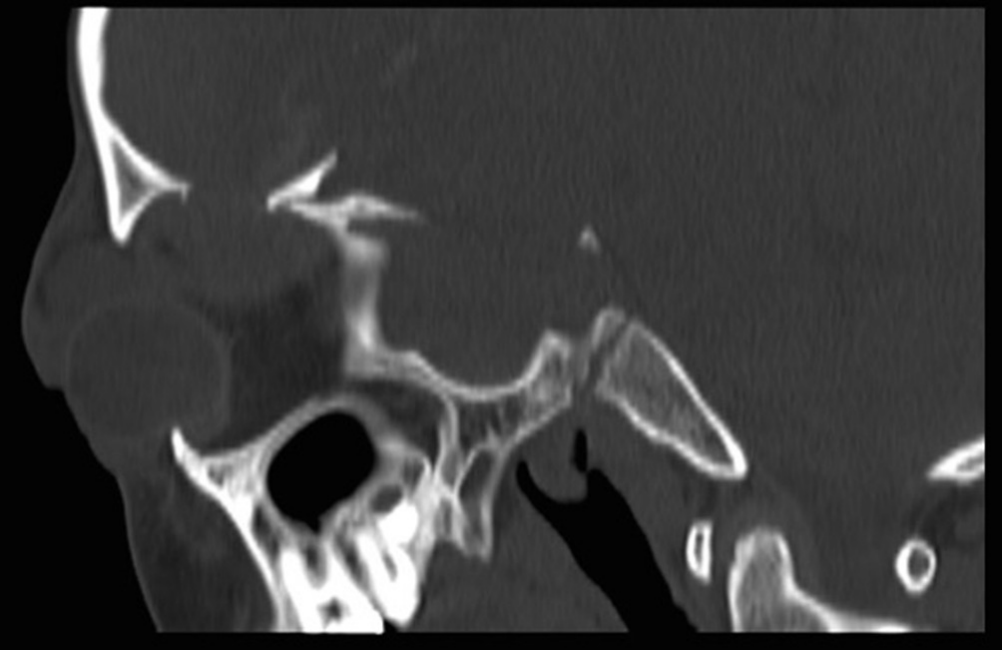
Sagittal reformatted CT bone window image showing the superoposteriorly displaced bone fragment.
